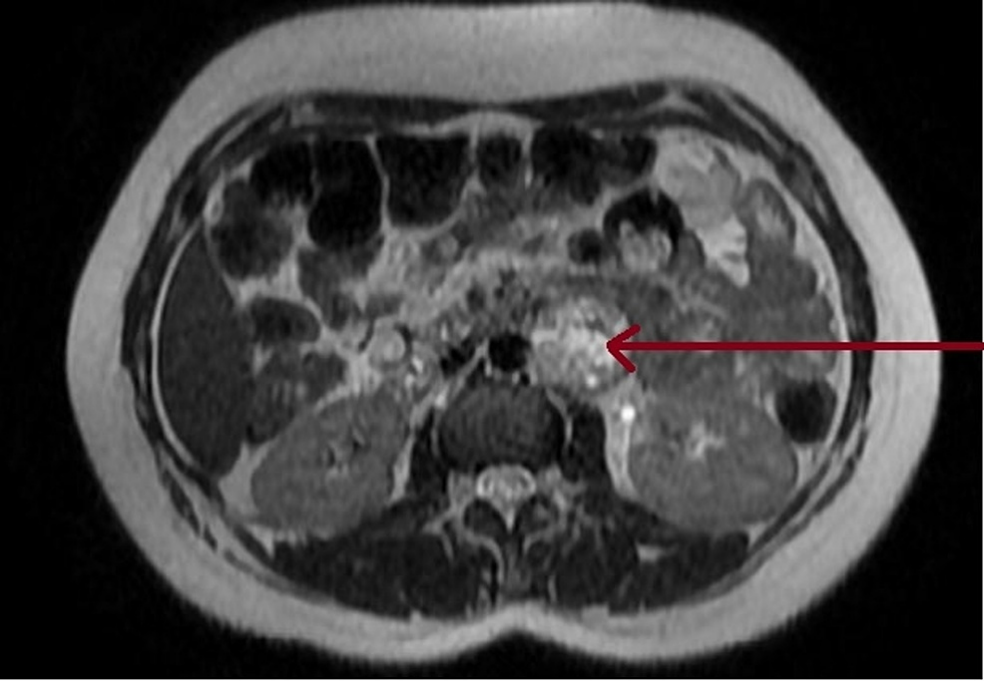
Abdominal MRI The image shows a left-lateralized abdominal juxta-aortic formation, well-limited, with heterogeneous T2 hyperintensity, and measuring 36 x 33 mm (red arrow) MRI: magnetic resonance imaging
